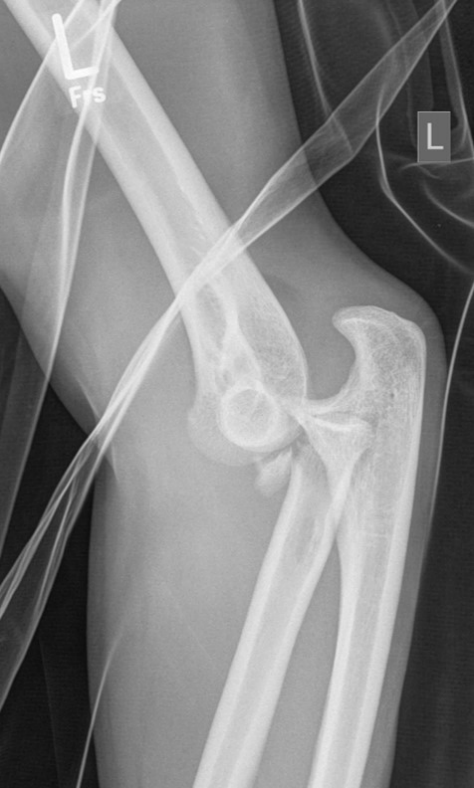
Female patient (36 years) with a Mason type III fracture of the radial head combined with a posterior elbow dislocation. Radiographs on the day of the injury.
